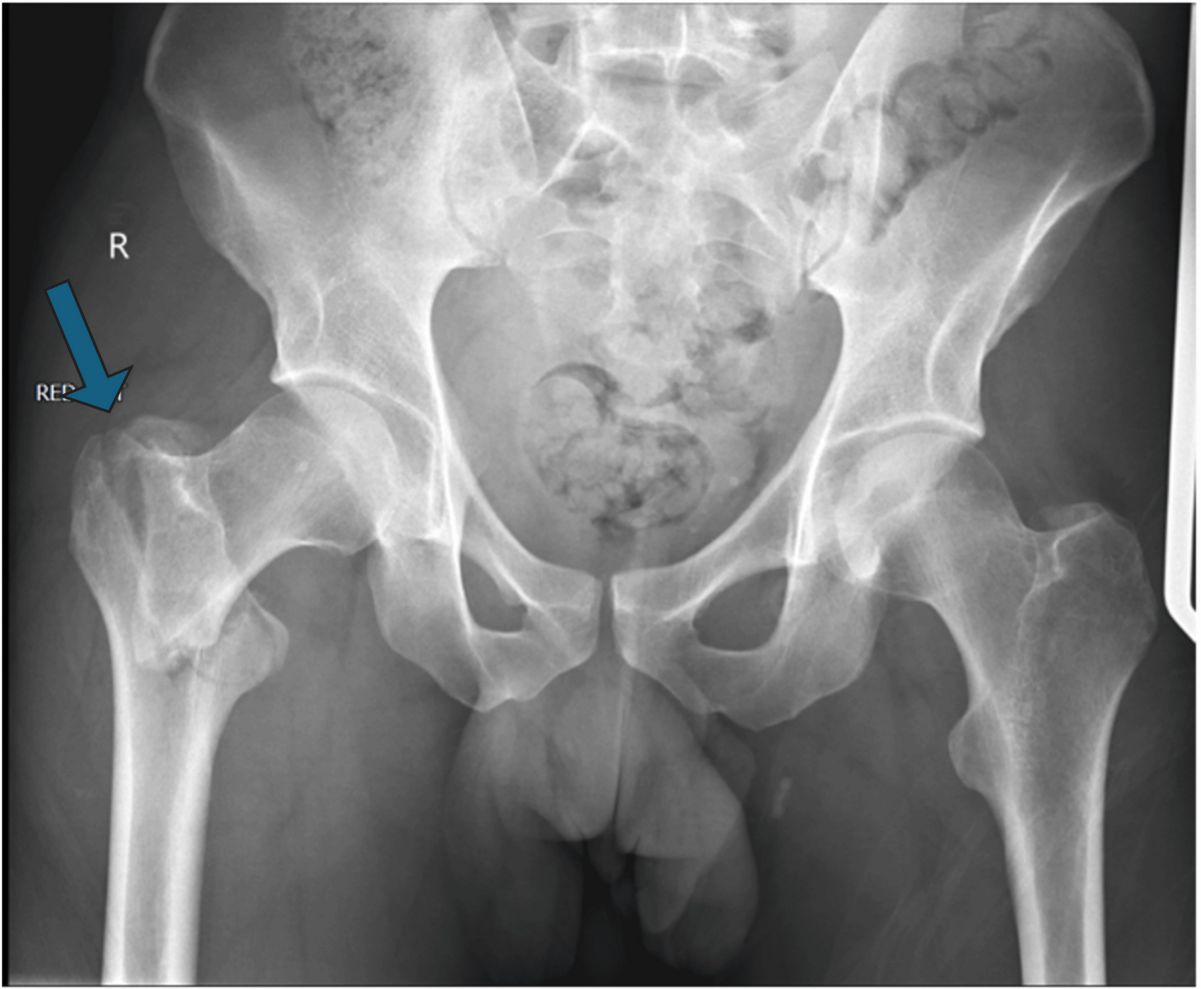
Plain radiograph of the pelvis showing a displaced right sided inter-trochanteric neck of femur fracture (arrow)
